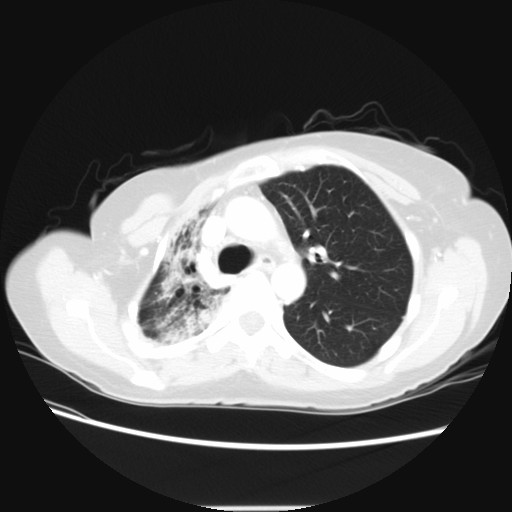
Chest CT obtained July 2007, approximately 1 year after pleurectomy with hyperthermic intraoperative intrapleural chemotherapy. An interstitial fibrosis is evident. Subsequent CT are largely unchanged but the patient's symptoms persist.
